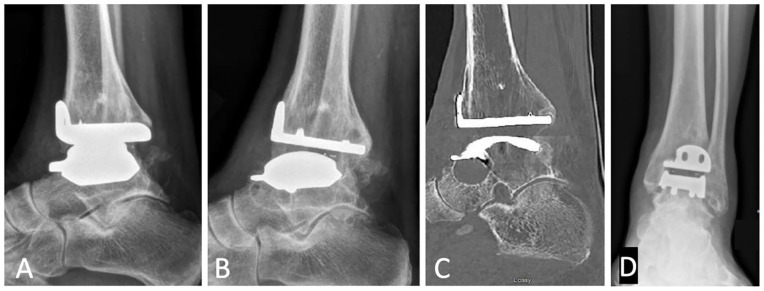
Imaging of patient with progressive subtalar cyst, (A) lateral radiograph postoperatively prior to cyst development, (B) lateral radiograph 6 years postoperatively, (C) sagittal computed tomography scan 6 years postoperatively, and (D) anteroposterior radiograph postoperatively with coronal malalignment.
